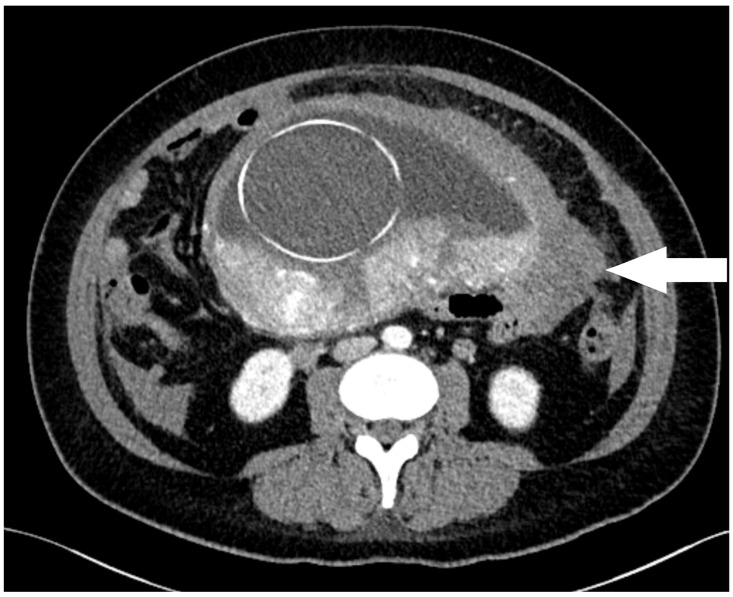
Contrast-enhanced computed tomography demonstrating hemoperitoneum along the left fundal region, with the arrow indicating the localized hematoma without evidence of uterine rupture or active extravasation.
